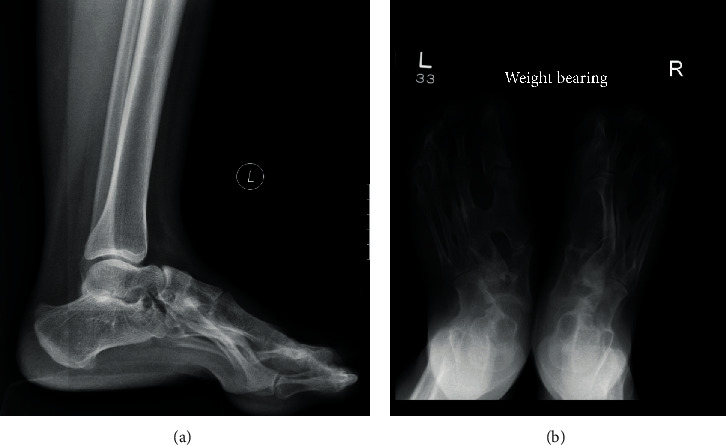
Companion case. 30-year-old man with the Apert syndrome. Lateral (a) and frontal (b) foot X-ray with multiple osseous synostosis involving both feet, including intermetatarsal coalition.
